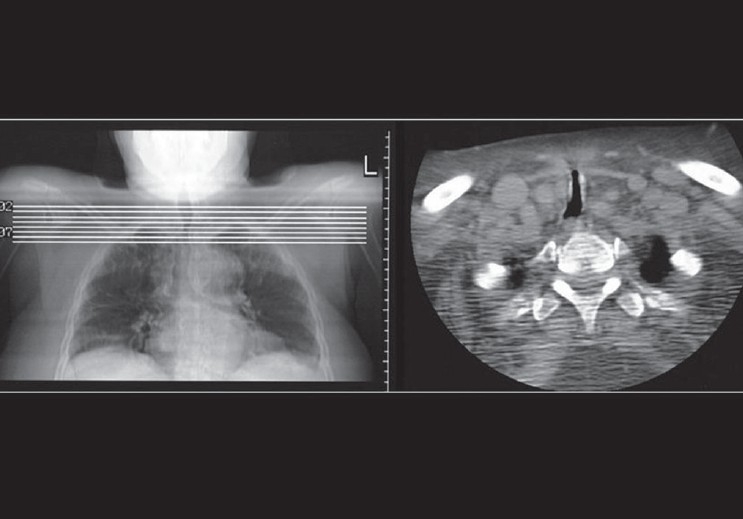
A scout film from a CT scan (left) shows narrowing of the trachea following prolonged ET placement. An axial CT confirms tracheal stenosis (right)
